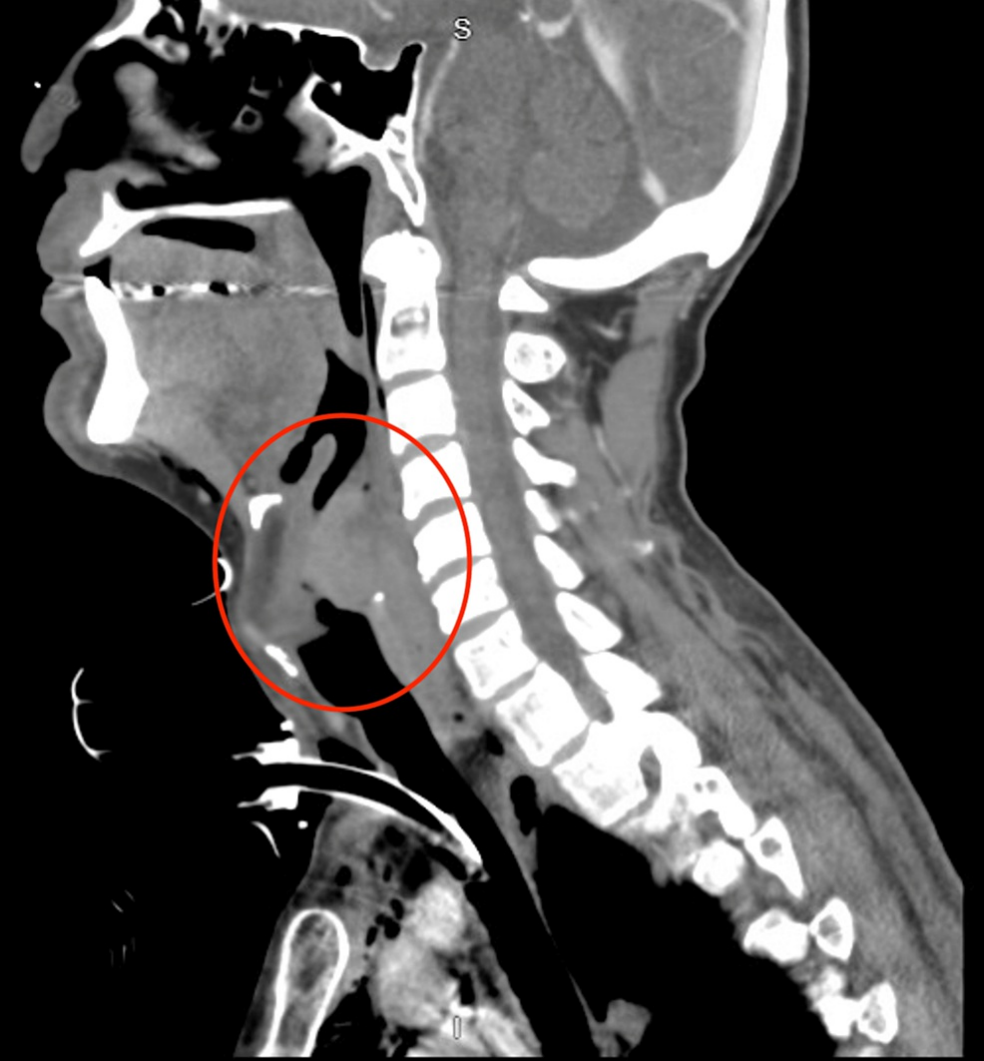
Soft tissue computed tomography found a large partially necrotic hypopharyngeal mass (red circle) measuring 4.8x4.4x3.3cm. Possibly originating from either retropharyngeal space or posterior mucosal space with involvement of aryepiglottic folds.
